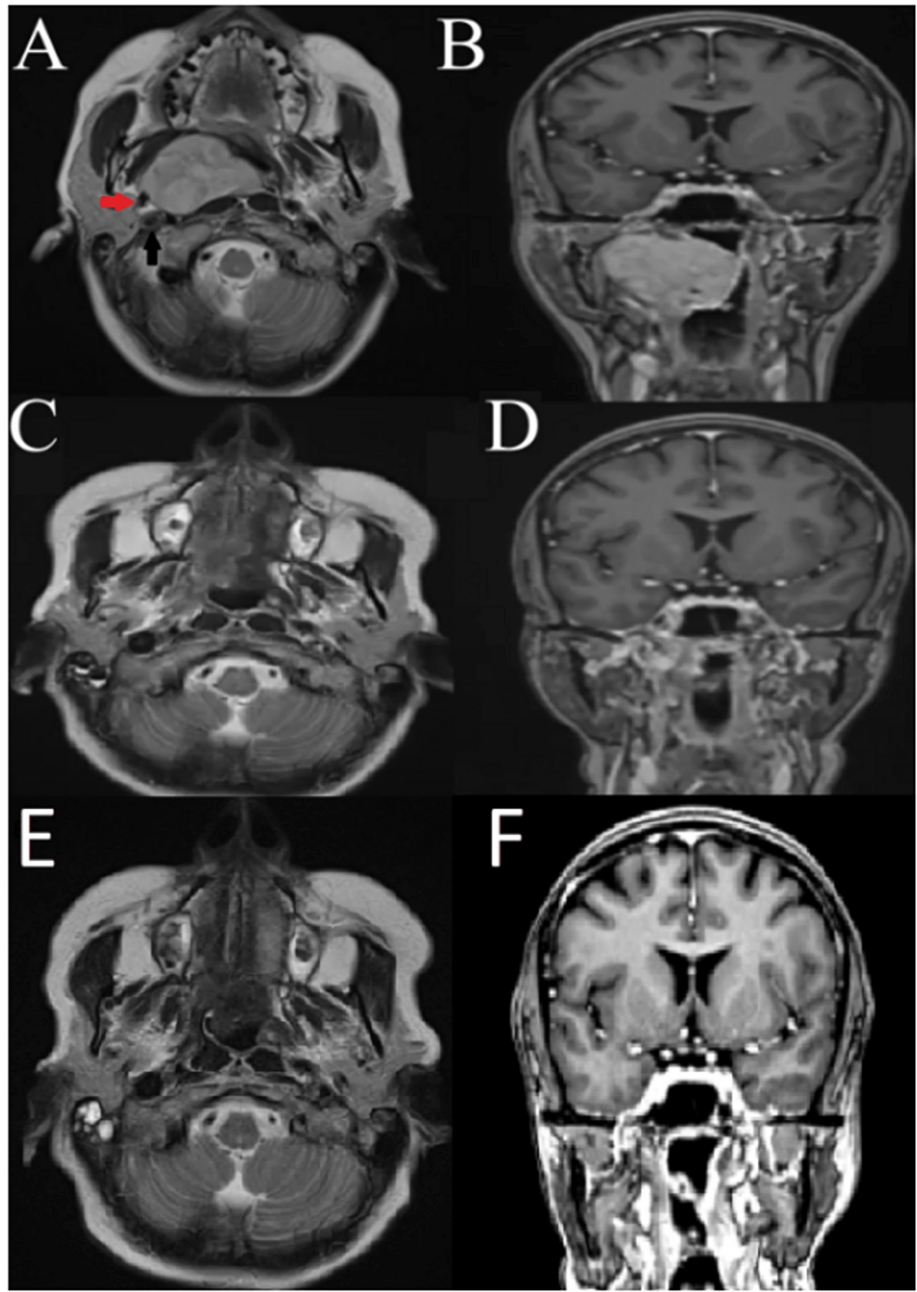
A: T2 axial MRI showing an expansive lesion in the right parapharyngeal space, laterally displacing the right common carotid artery (red arrow) and right internal jugular vein (black arrow). B: T1 with contrast coronal MRI showing an expansive lesion in the right parapharyngeal space. C, D: Axial and coronal MRI three months after lesion resection; E, F: Axial and coronal MRI 12 months after lesion resection.
